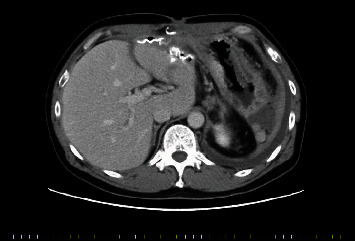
Abdominal computed tomography shows a perihepatic abscess around foreign bodies with air bubbles 5 months after the initial operation.
